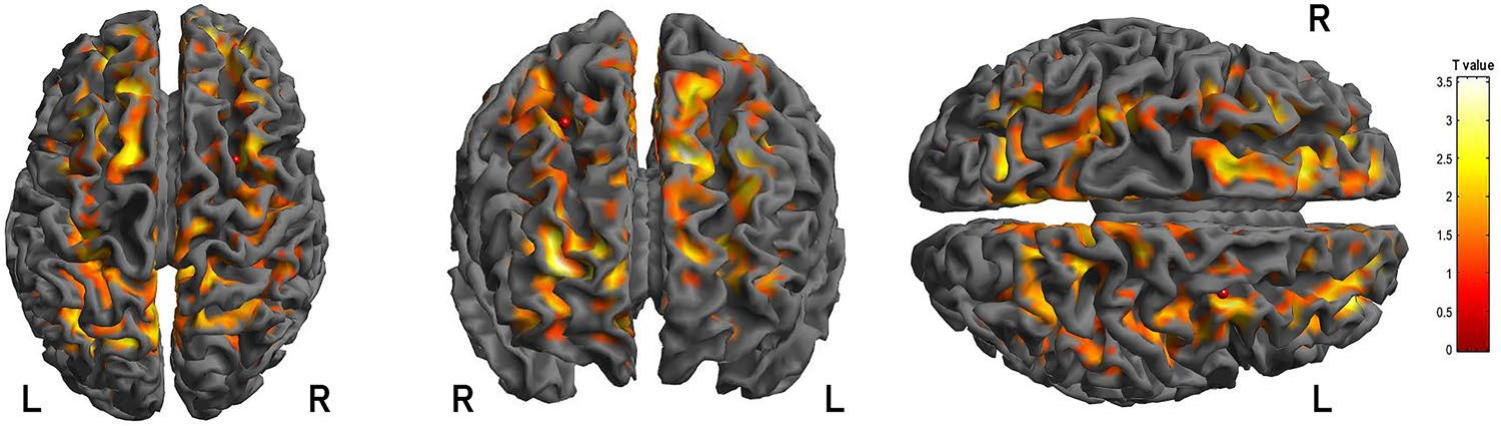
3D rendering showing the results of SPM comparisons between 18 F-FDG uptake in patients OSA patients undergoing the longitudinal evaluation. At the 12-month follow-up, a significant increase of cortical glucose consumption is detectable in right and left frontal and parietal cortex and in in OSA patients. R: right; L: left
